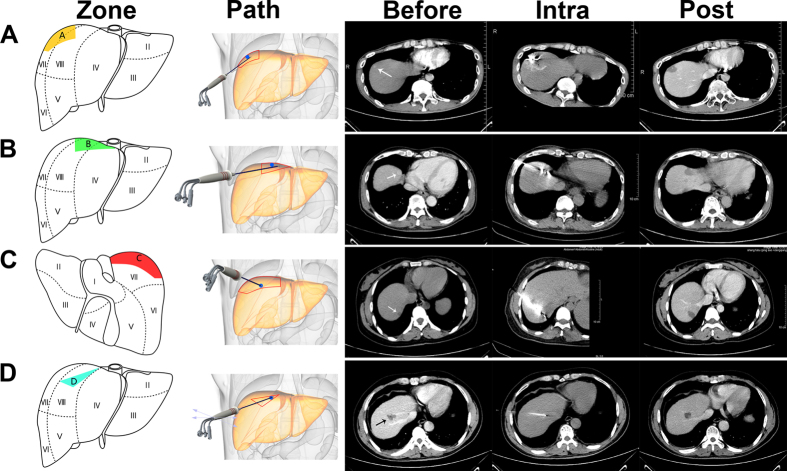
Different puncture paths for ablating nodules at various positions. (A) A recurrent small HCC in zone A (arrowhead) was found in a 36-year-old male patient who underwent TACE treatment 8 months previously; no viable tumor was found in the 4-year postoperative CT scan image. (B) a recurrent small HCC in zone B (arrowhead) was found in a 61-year-old male patient who underwent hepatectomy 4 years previously; no viable tumor was found in the 2-year postoperative CT scan image. (C) a recurrent small HCC in zone C (arrowhead) was found in a 42-year-old female patient who underwent hepatectomy 1 year previously; no viable tumor was found in the 1-year postoperative CT scan image. (D) a recurrent small HCC in zone D (arrowhead) was found in a 52-year-old male patient who underwent TACE treatment 3 months previously; no viable tumor was found in the 6-month postoperative CT scan image.
